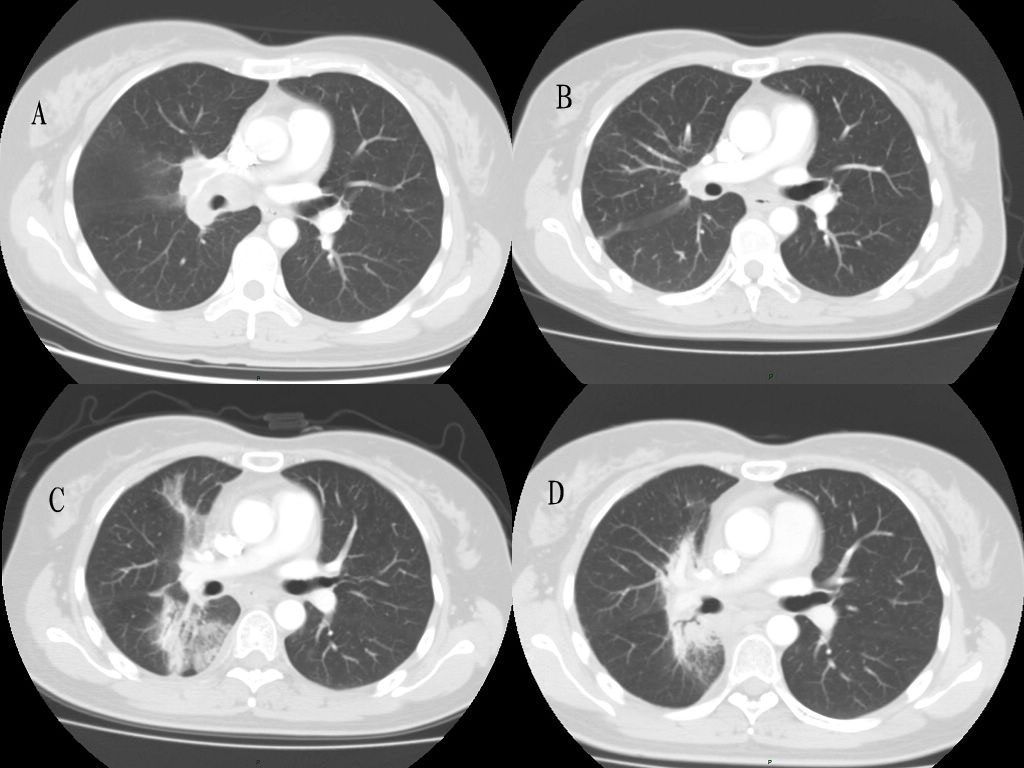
Thoracic CT scans of Patient 10 (A) before radiotherapy, (B) one month after end of radiotherapy, (C) 4 months after end of radiotherapy when RRP took place induced by consolidation chemotherapy, and (D) days after application of systematic steroids. (A)(B): No pulmonary infiltrate, (C): Pulmonary ground-glass opacity, (D): Partial resolution of the lung infiltrate.
